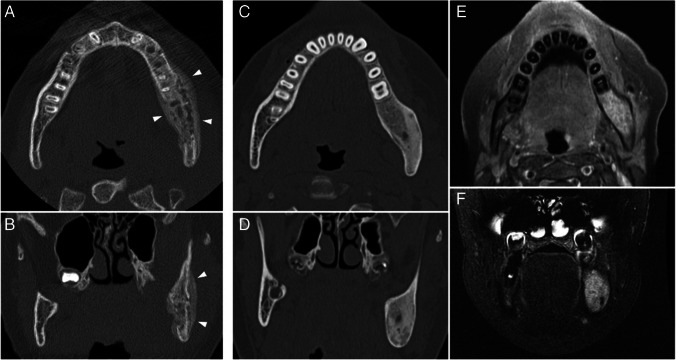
Evolution of mandibular lesions: images obtained from a boy at ages 12 (a, b) and 13 (c–f) years. a, b Axial (a) and coronal (b) computed tomography (CT) images of the mandible show an active inflammatory process in the left body and ramus, with a heterogeneous pattern, predominantly lytic, with cortical erosion, and with periosteal reaction (*arrowheads*). c, d Axial (c) and coronal (d) CT images of the same patient 1 year later show the inflammatory process has partially resolved, and that the bone is now expanded but conserves its cortical borders, showing a more diffuse ground-glass density. e, f Axial T1-weighted contrast-enhanced fat-saturated (e) and T2-weighted fat-saturated (f) magnetic resonance images reveal there are still signs of inflammation, with contrast enhancement and bone edema seen on (e) and (f), respectively
